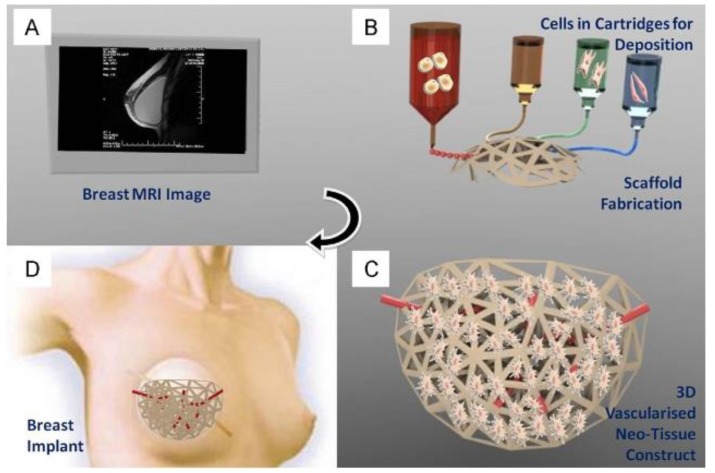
Application of patient’s MRI data to generate a bioprinted scaffold for organ regeneration, disease treatment, or drug delivery. MR images (A) of the target organ/tissue will be acquired and processed to create a 3D STL file. The model will be 3D bioprinted using various inks and scaffolds (B), cultured in vitro to establish the new tissue structure and vasculature (C), followed by implantation in vivo to repair/regenerate target tissue/organ (D). Reproduced with permission from Ref. [19].
